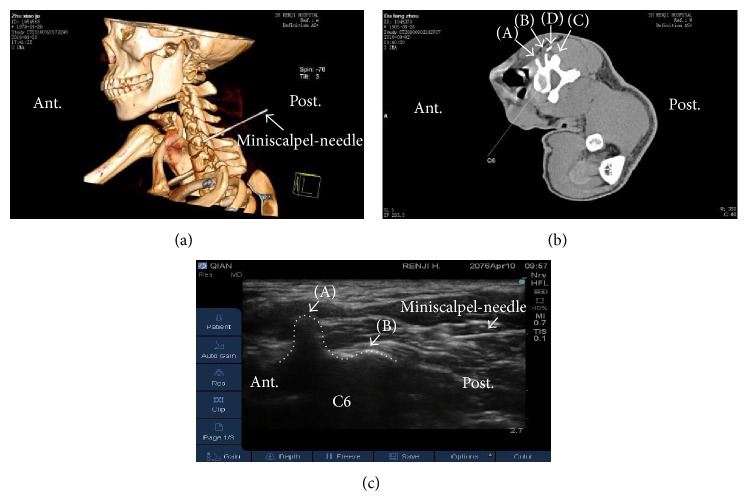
UG-MSN release at the sixth cervical vertebra (C6). (a) Three-dimensional computed tomography reconstruction of the puncture site at C6. The white arrow indicates the MSN. (b) Conventional computed tomography scanning of C6. The letters (A)–(D) indicate, respectively, the anterior tubercle, posterior tubercle, articular process, and the MSN. (c) UG-MSN release at C6, with the MSN clearly indicated. Trigger points showed the following characteristics: hyperechoic skin, adipose tissue of mixed echogenicity, and muscle of hyperechoic, marbled appearance. The dotted line shows the outline of C6. The letters (A) and (B) represent, respectively, the posterior tubercle and articular process. Post.: posterior position of patients. Ant.: anterior position of patients.
